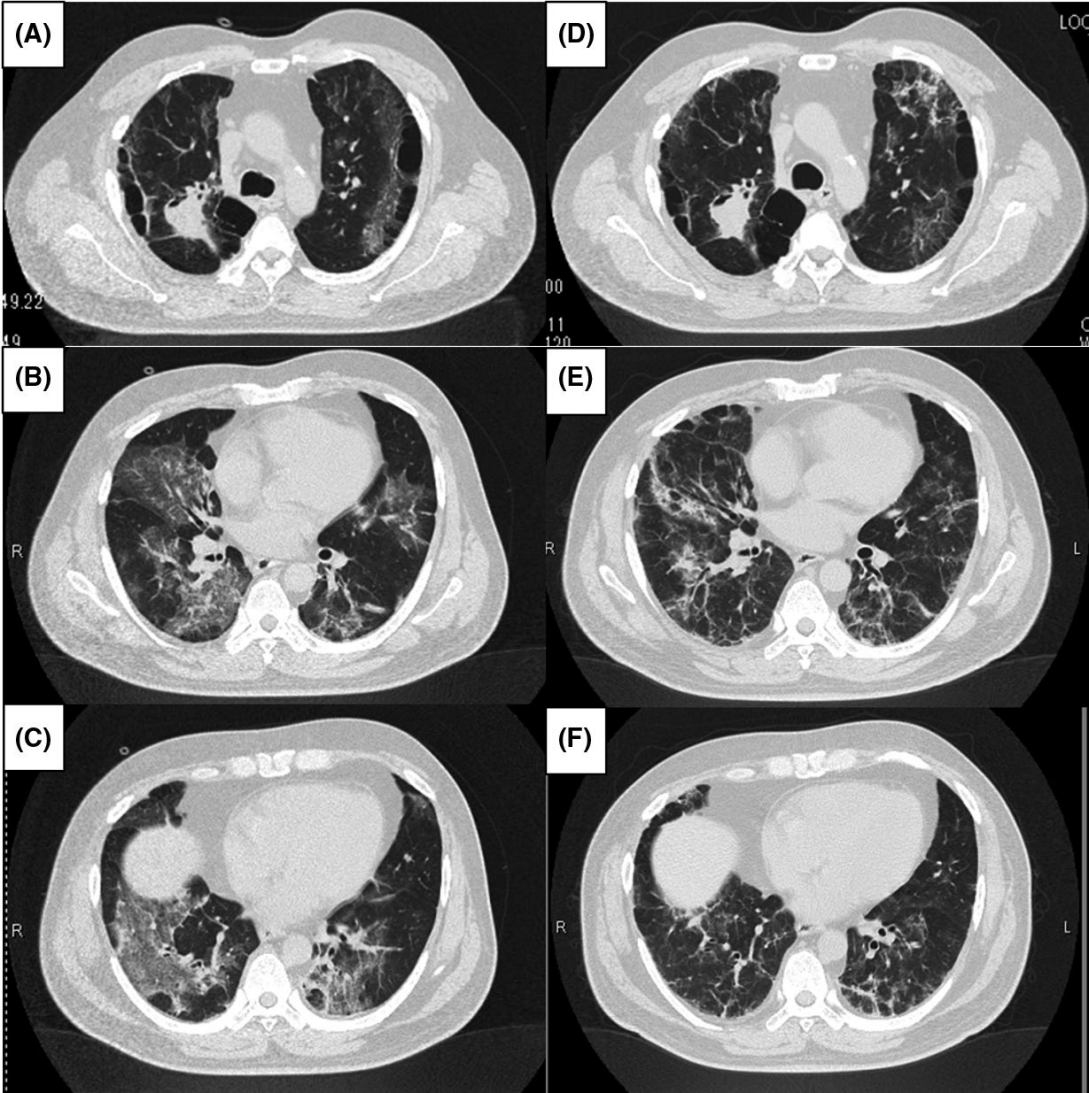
Chest computed tomography showing diffuse ground‐glass opacities in both lungs on the 6th day of COVID‐19 onset (A‐C). Chest computed tomography revealing that ground‐glass opacities in both lungs reduced, but linear and reticular shadows remained on the 29th day of COVID‐19 onset (D‐F)
